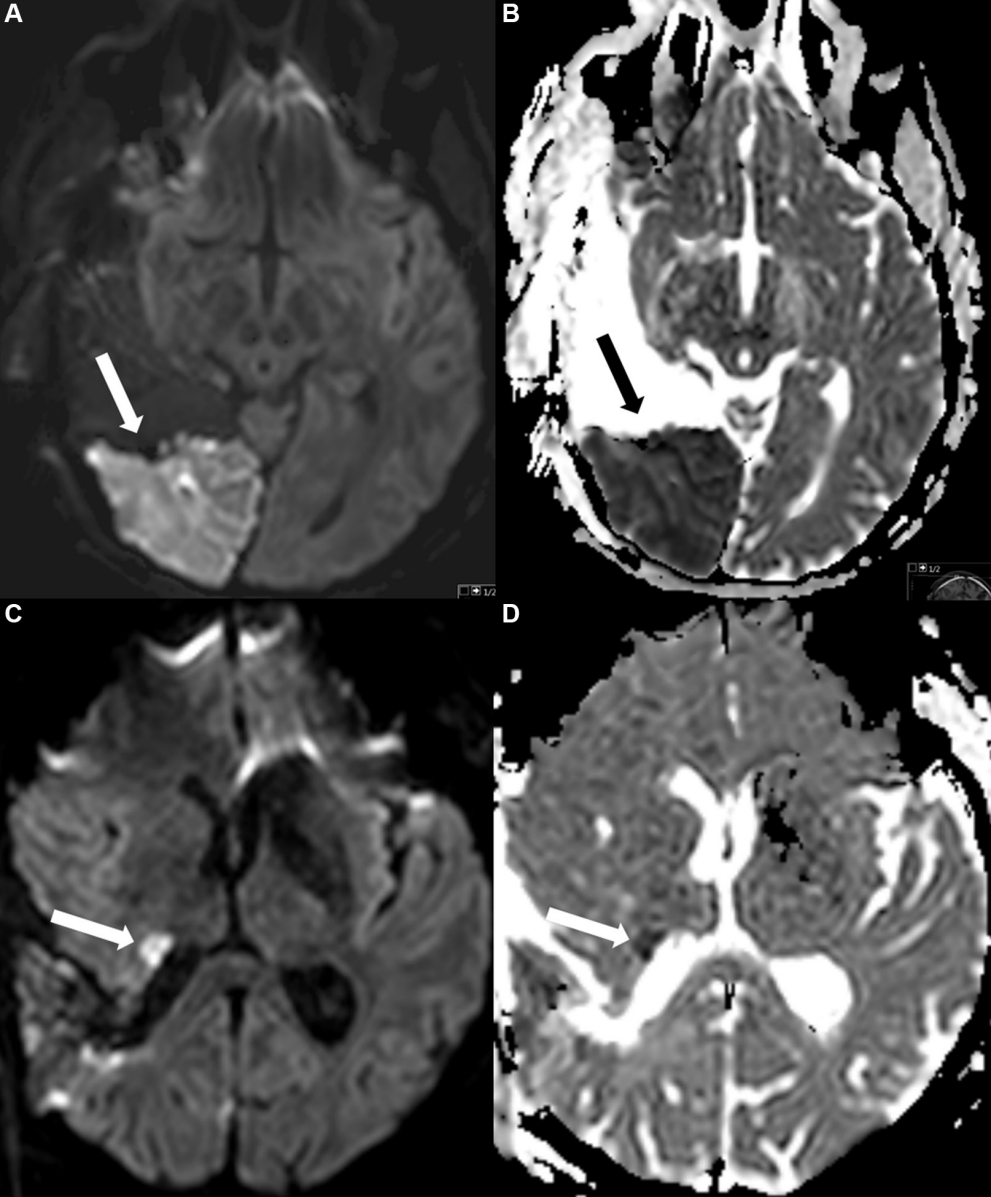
Examples of postoperative ischemic changes The first row (Figure A and B) shows a large area with restricted diffusion (A) and corresponding hypointensity in the ADC map (B), the second row a smaller area with restricted diffusion (DWI) (C) and ADC (D) map.
